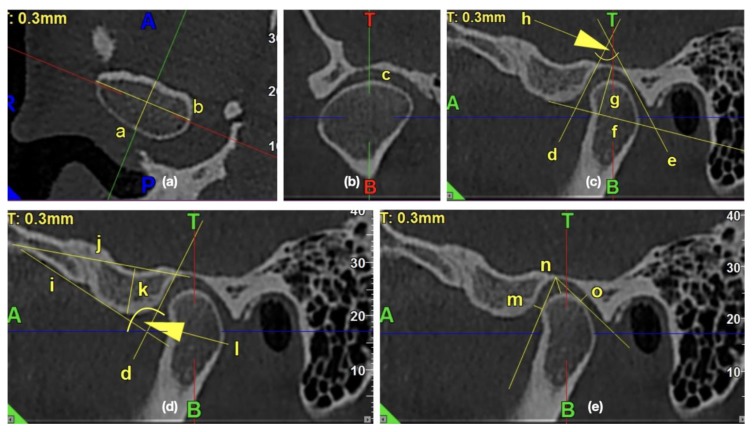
The exemplary lines, points, and angles in the temporomandibular joints (TMJ) cone beam computed tomography (CBCT) scans used for measurements. (a) Morphology of mandibular condyle in the axial view: /a/condylar A-P dimension, /b/condylar width; (b) Shape of condyle head in the coronal view: /c/condyle’s superior surface view assessment; (c) Morphology of glenoid fossa in the sagittal view: /d/PE line, /e/AT line, /f/glenoid fossa basal line, /g/glenoid fossa depth, /h/glenoid fossa divergence angle; (d) Morphology of articular eminence in the sagittal view: /i/AE line, /j/eminence basal line, /k/articular eminence height, /l/articular eminence divergence angle; (e) Assessment of anterior, posterior, and superior joint spaces in the sagittal view: /m/anterior joint space, /n/superior joint space, /o/posterior joint space.
